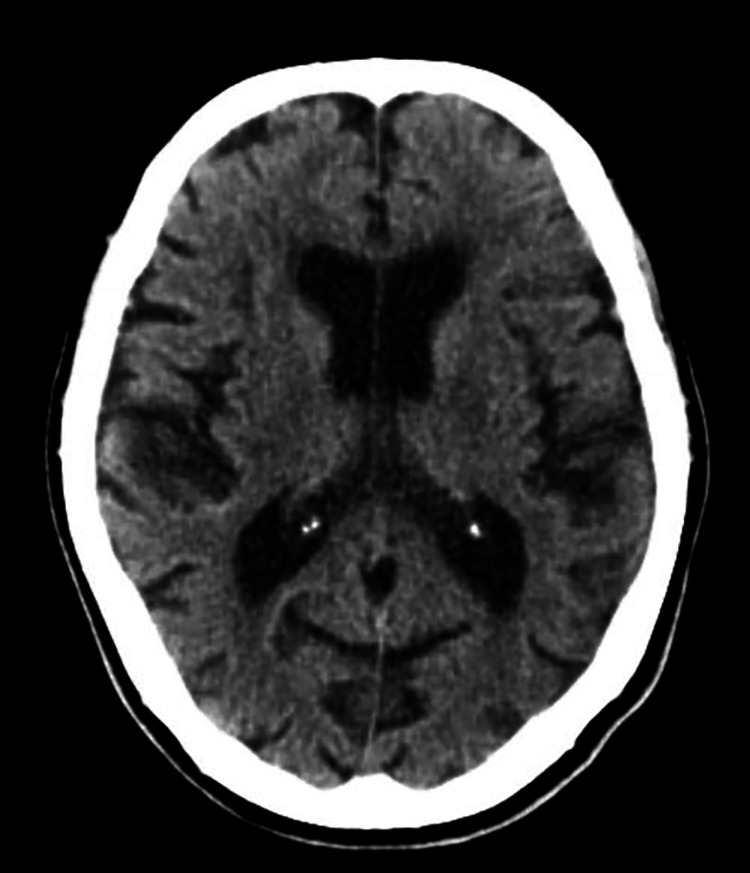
CT head without contrast. CT head without contrast showed moderate diffuse atrophy and no acute intracerebral changes, hemorrhage, or infarcts.
